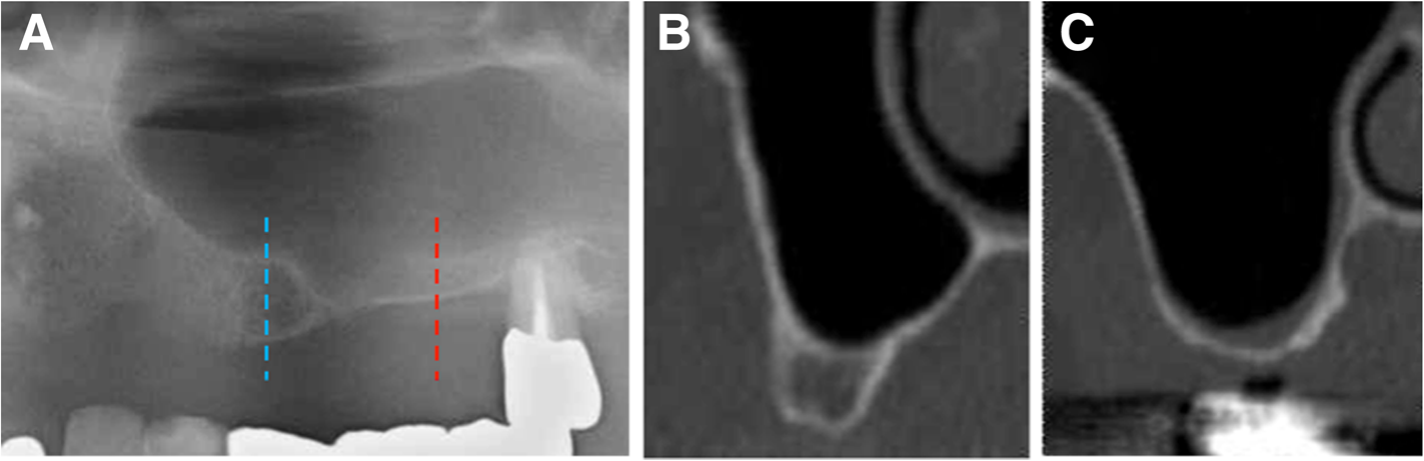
Preoperative radiographic images. a Orthopantomography shows that inadequate bone height was observed in sites #24 (*blue line*) and #26 (*red line*). b Coronal CT image of the *blue line*; vertical bone height is 4 mm. c Coronal CT image of the *red line*; vertical bone height is only 1 mm
